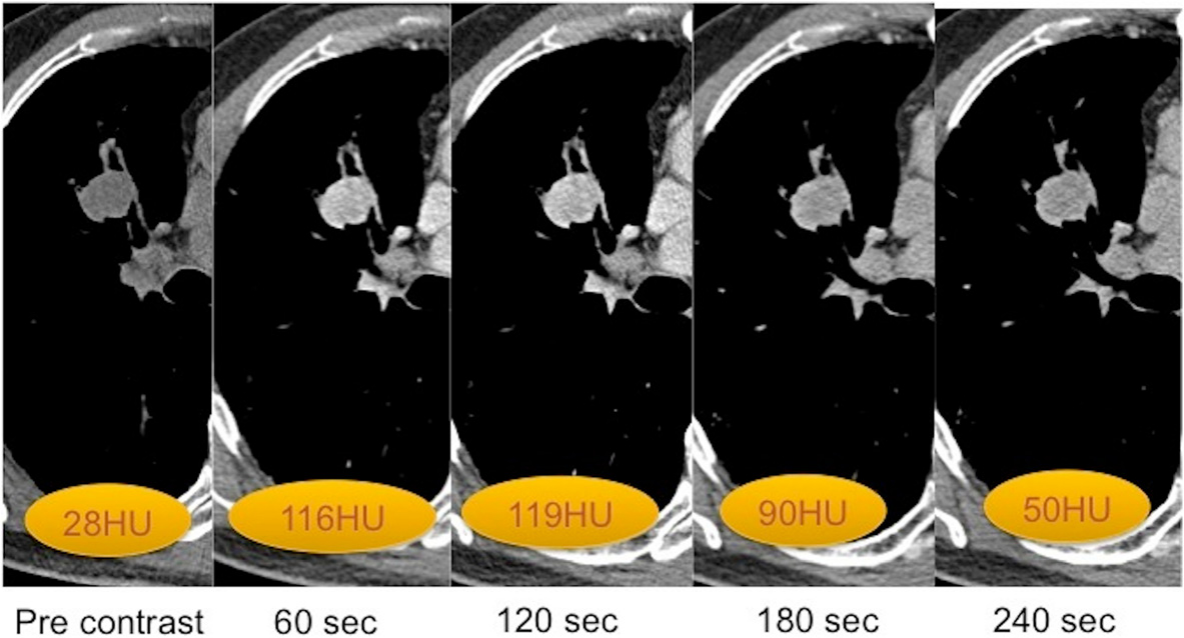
Right upper lobe Carcinoid tumour: Series of axial DCE-CT slices that demonstrates a hyperenhancing lobulated endobronchial nodule with a mean CT enhancement (HU) number 91. CT guided biopsy confirmed a typical carcinoid tumour
